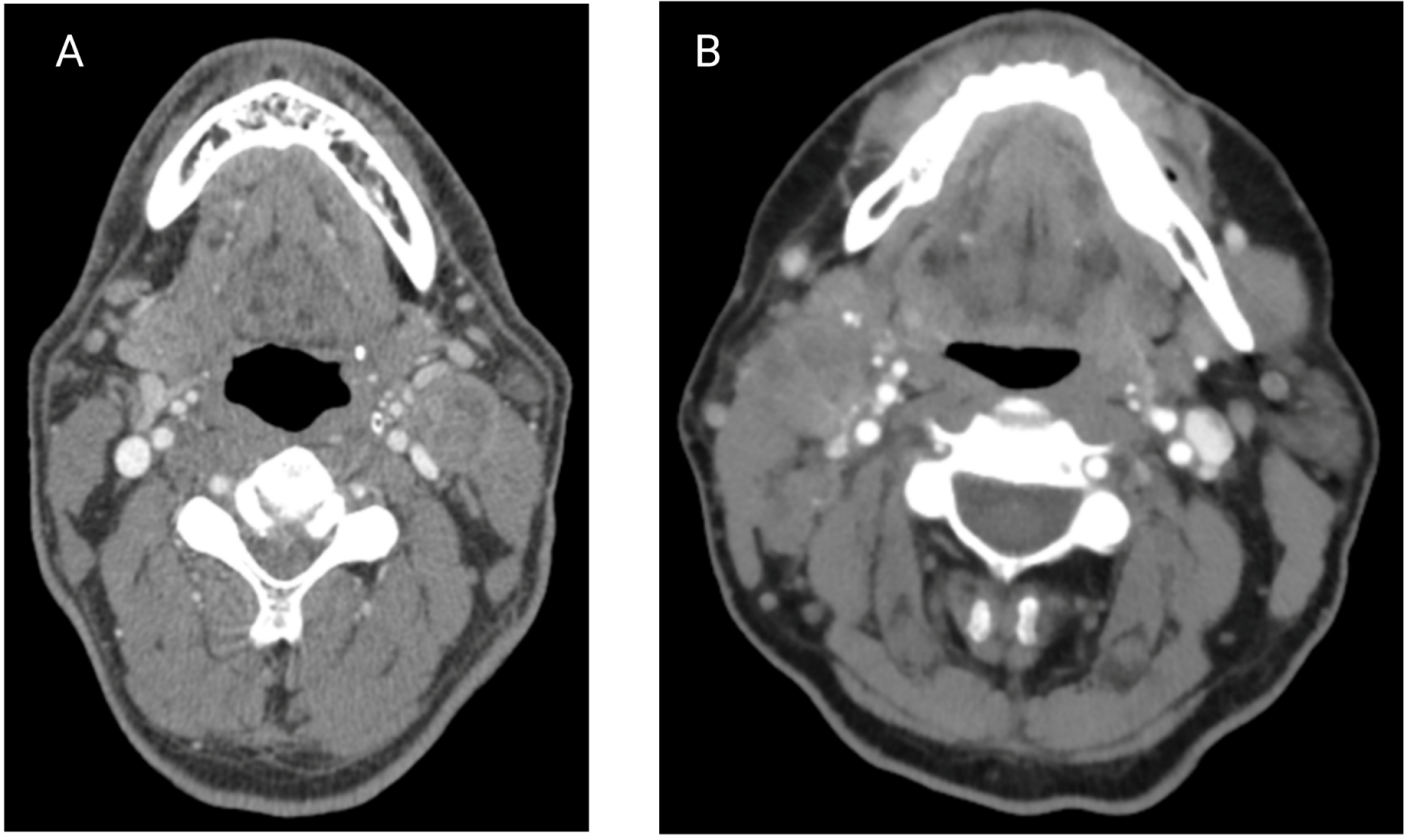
(A) Contrasted axial CT scan of a patient with a L level II node suspicious for iENE, which was later confirmed pathologically. (B) Contrasted axial CT scan of a patient with a R level II node suspicious for iENE, but ENE was not found on pathology. (Images kindly provided by Dr. James Bates).
